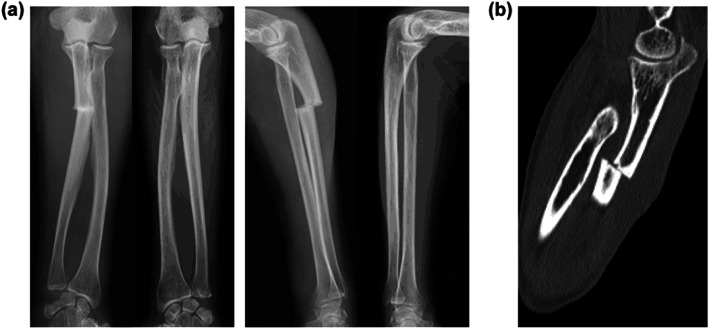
a Preoperative radiography of the bilateral forearm; b preoperative computed tomography. Sclerotic changes are seen at the fracture site
